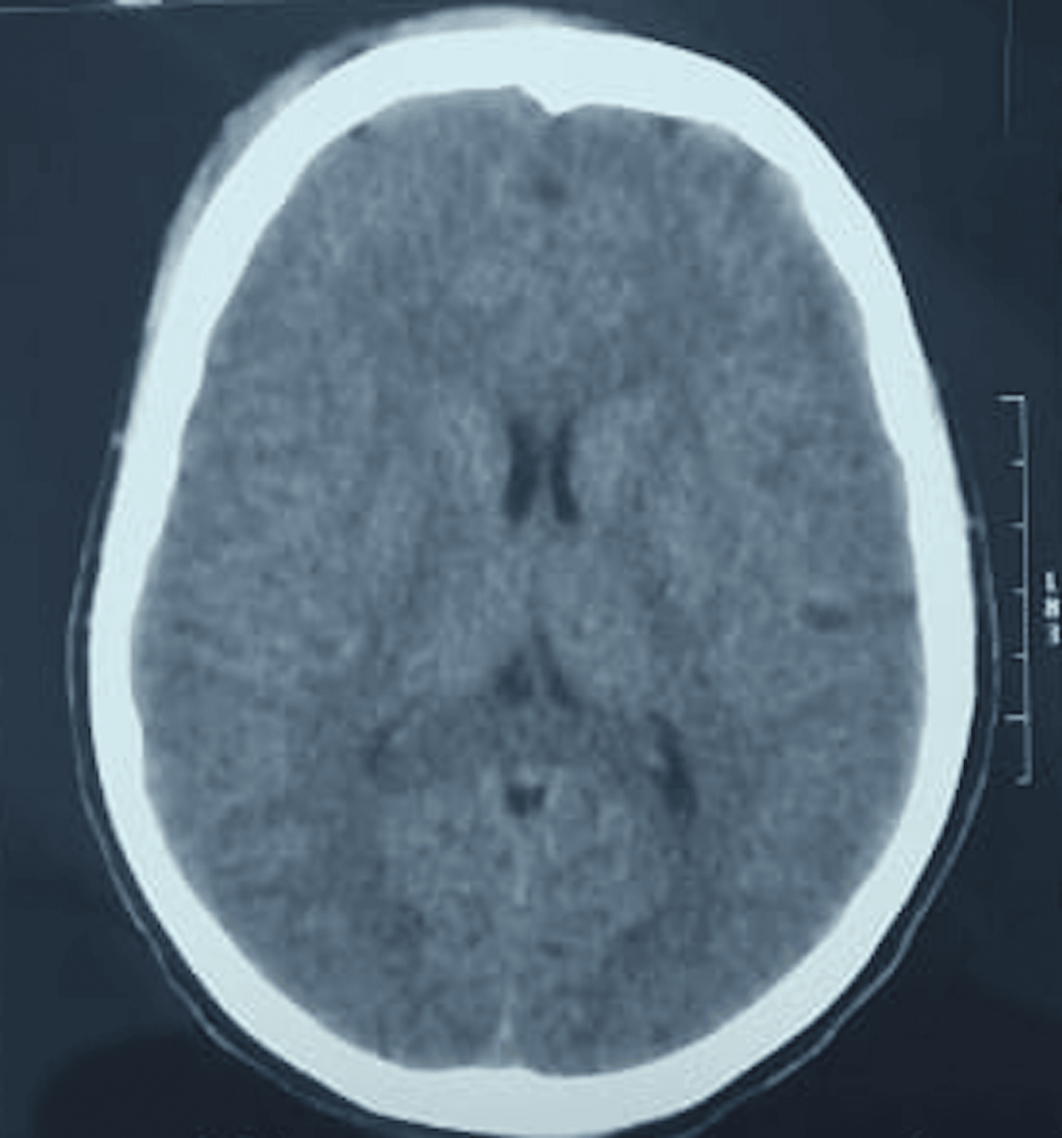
A CT scan of brain shows no residual parenchymal lesion.
